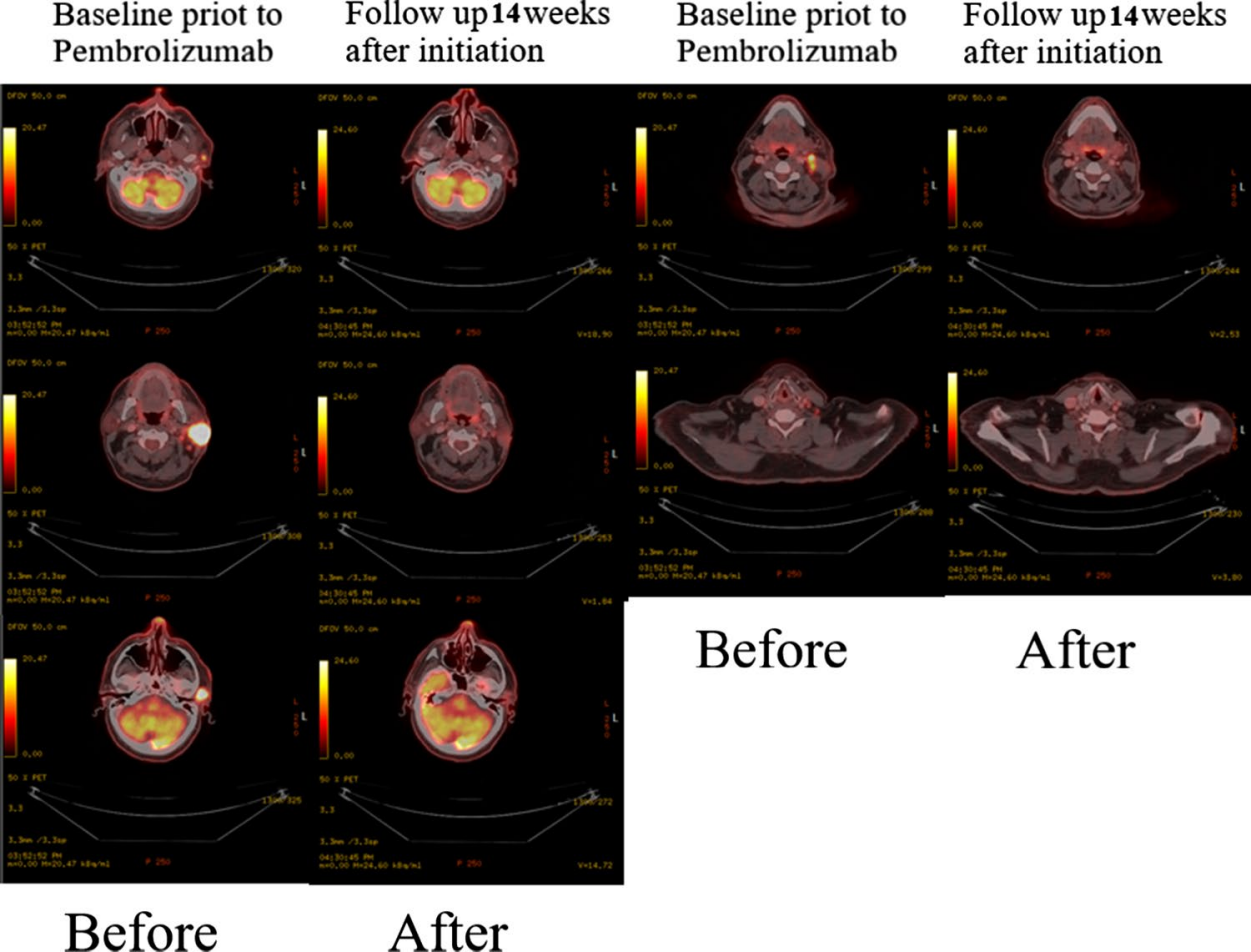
PET CT before and after treatment. Left side column—baseline prior to Pembrolizumab. Right side column—follow up 14 weeks after initiation
